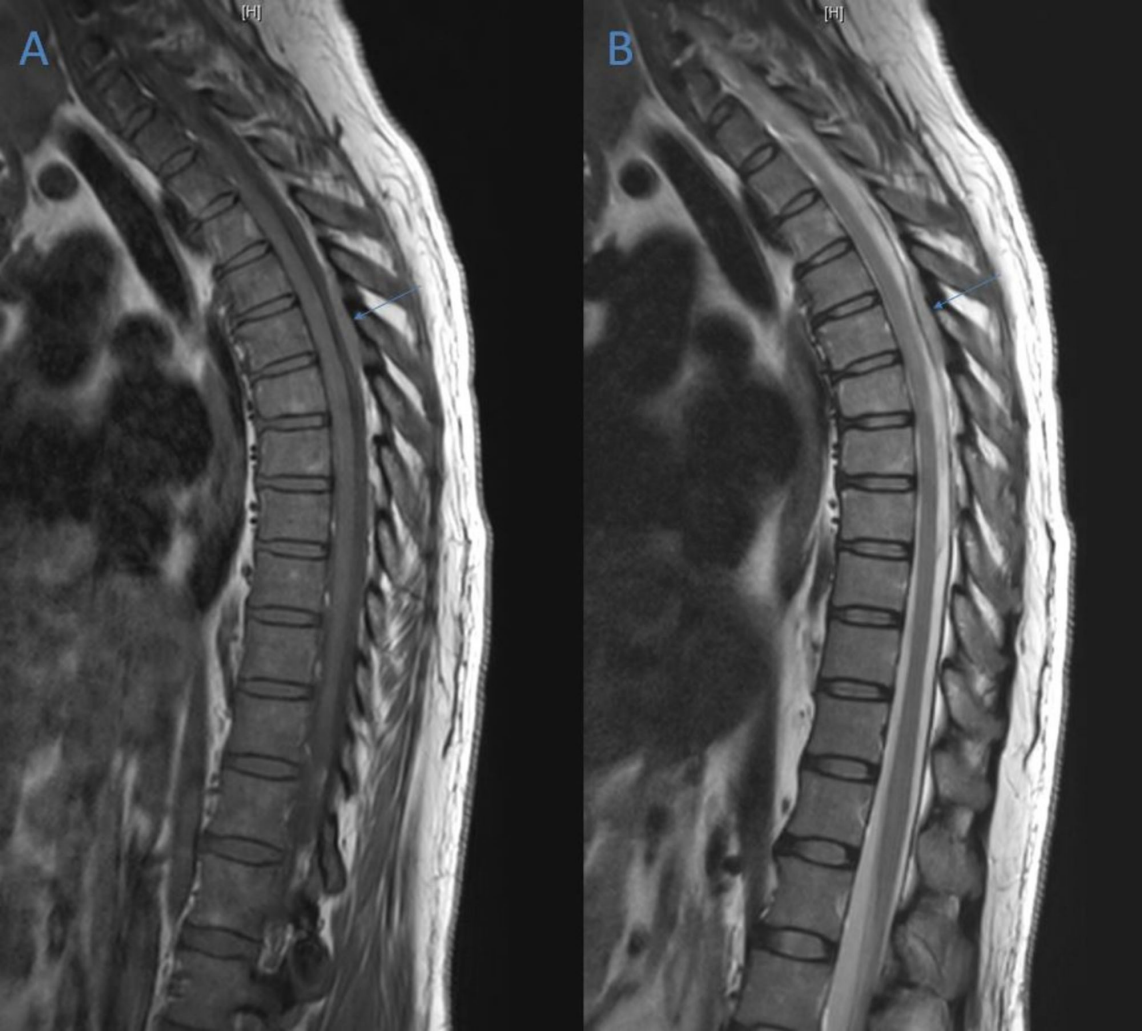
Sagittal Magnetic Resonance Imaging (MRI) from Day Eight of Admission. (A) Gadolinium-enhanced T1-weighted sagittal MRI showing a decrease in size of epidural T5 lesion (arrow). (B) Gadolinium-enhanced T2-weighted sagittal MRI showing a decrease in size of epidural T5 lesion (arrow).
